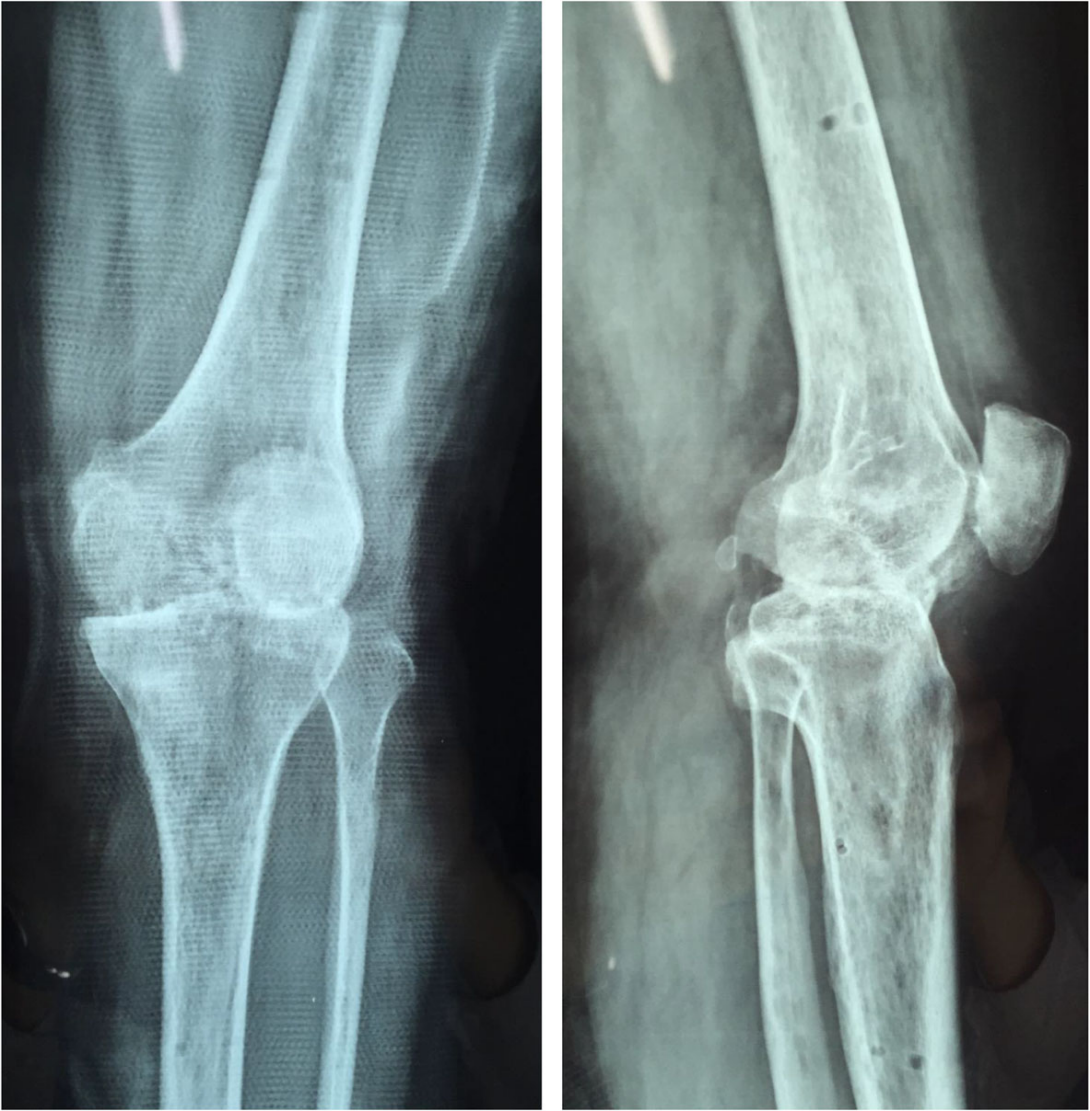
X-ray (anteroposterior and lateral): good knee fusion after removal of the external fixator (8.5 months after surgery)
